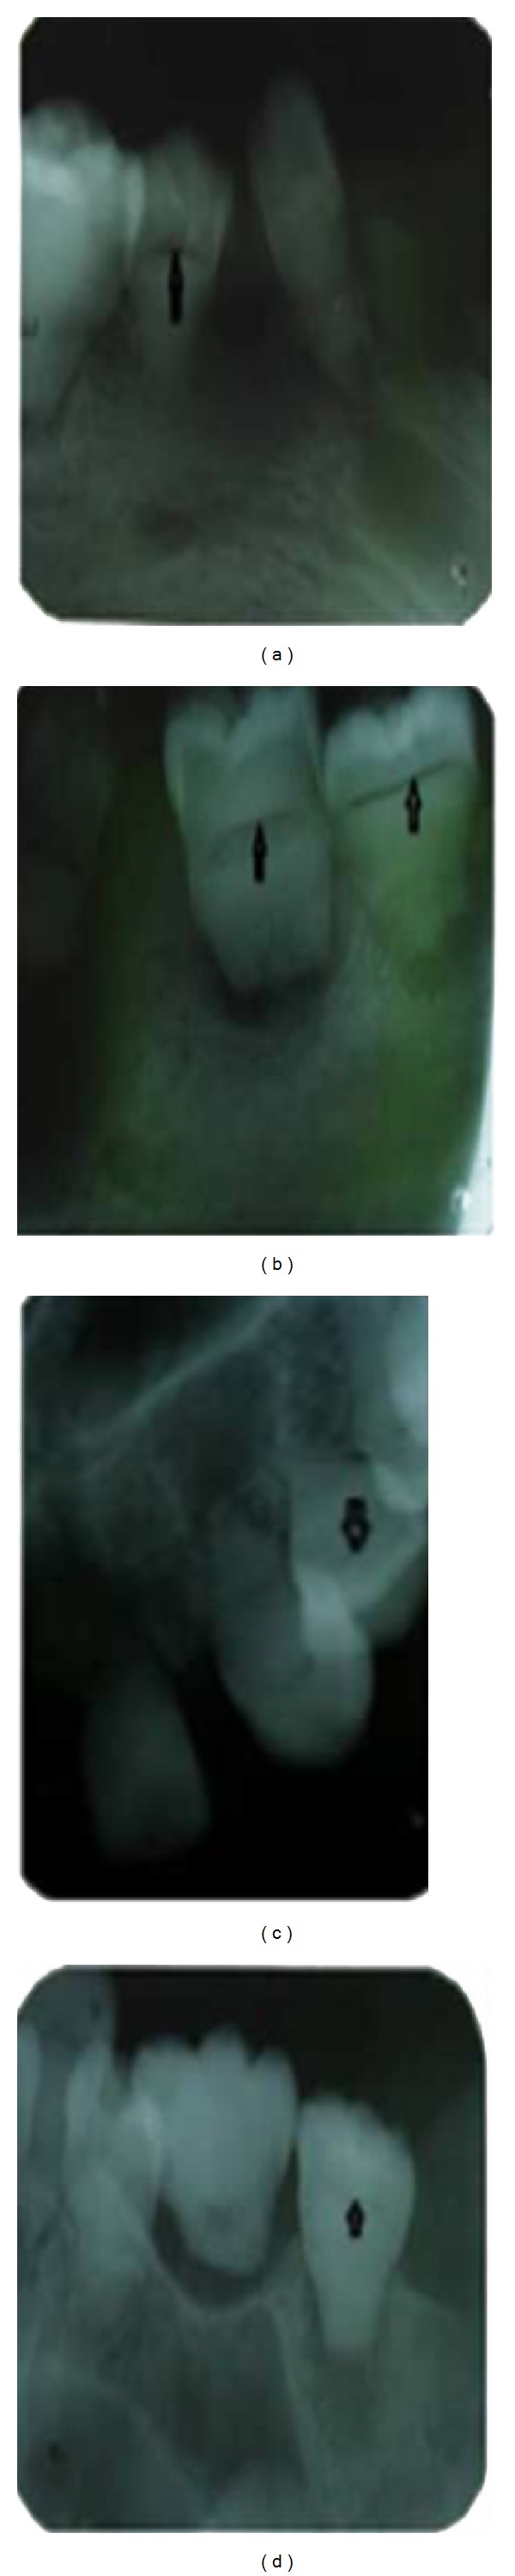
Intraoral periapical radiographs showing horizontal/crescent shaped pulpal remnants in pulp chambers (black arrows).
